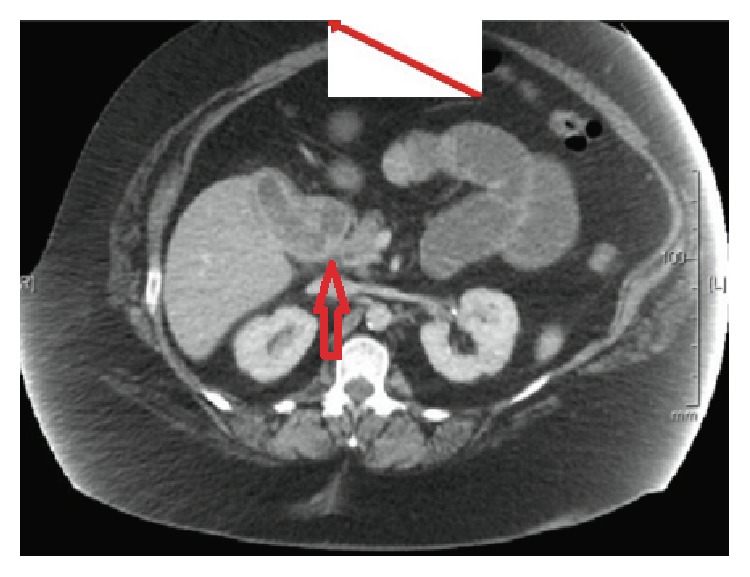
Contrast CT scan depicting cholecystoduodenal fistula (short red arrow).
